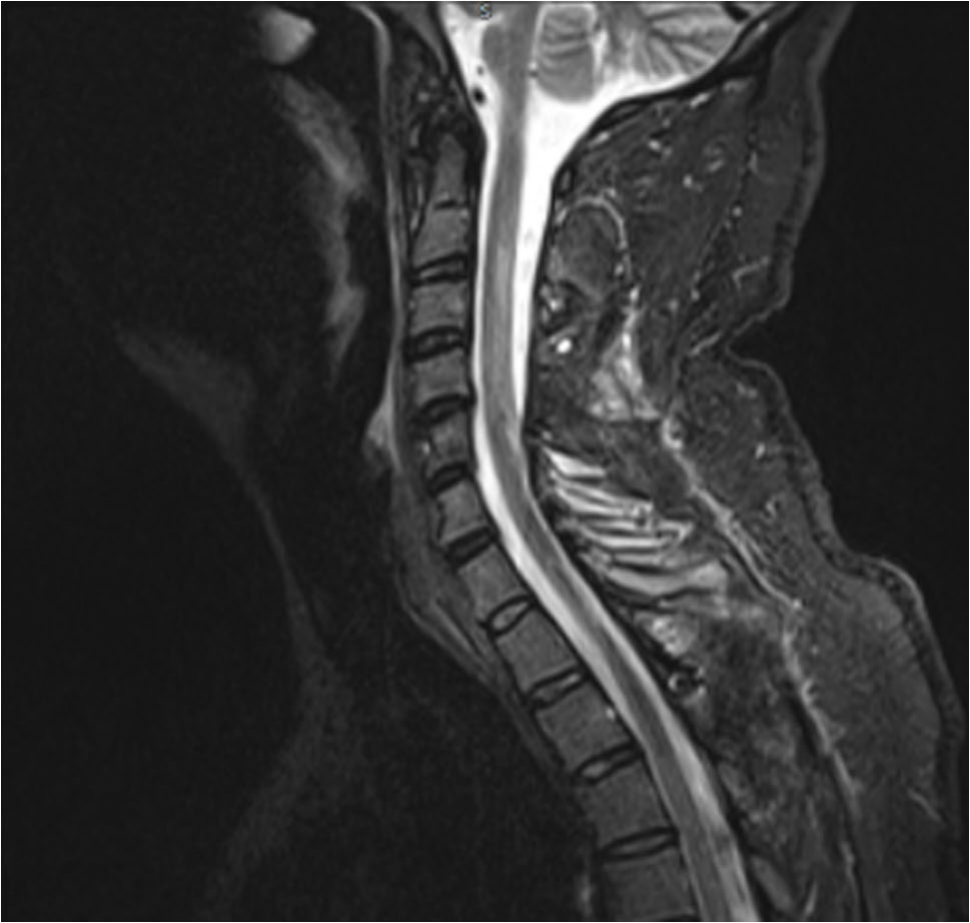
T2-weighted MRI scan 14 weeks post re-exploration following tension pneumosyrinx
